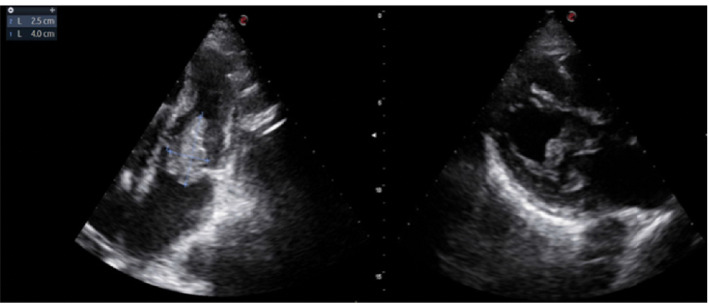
Transthoracic echocardiogram showing 2.5 × 4 cm mitral valve vegetation.
